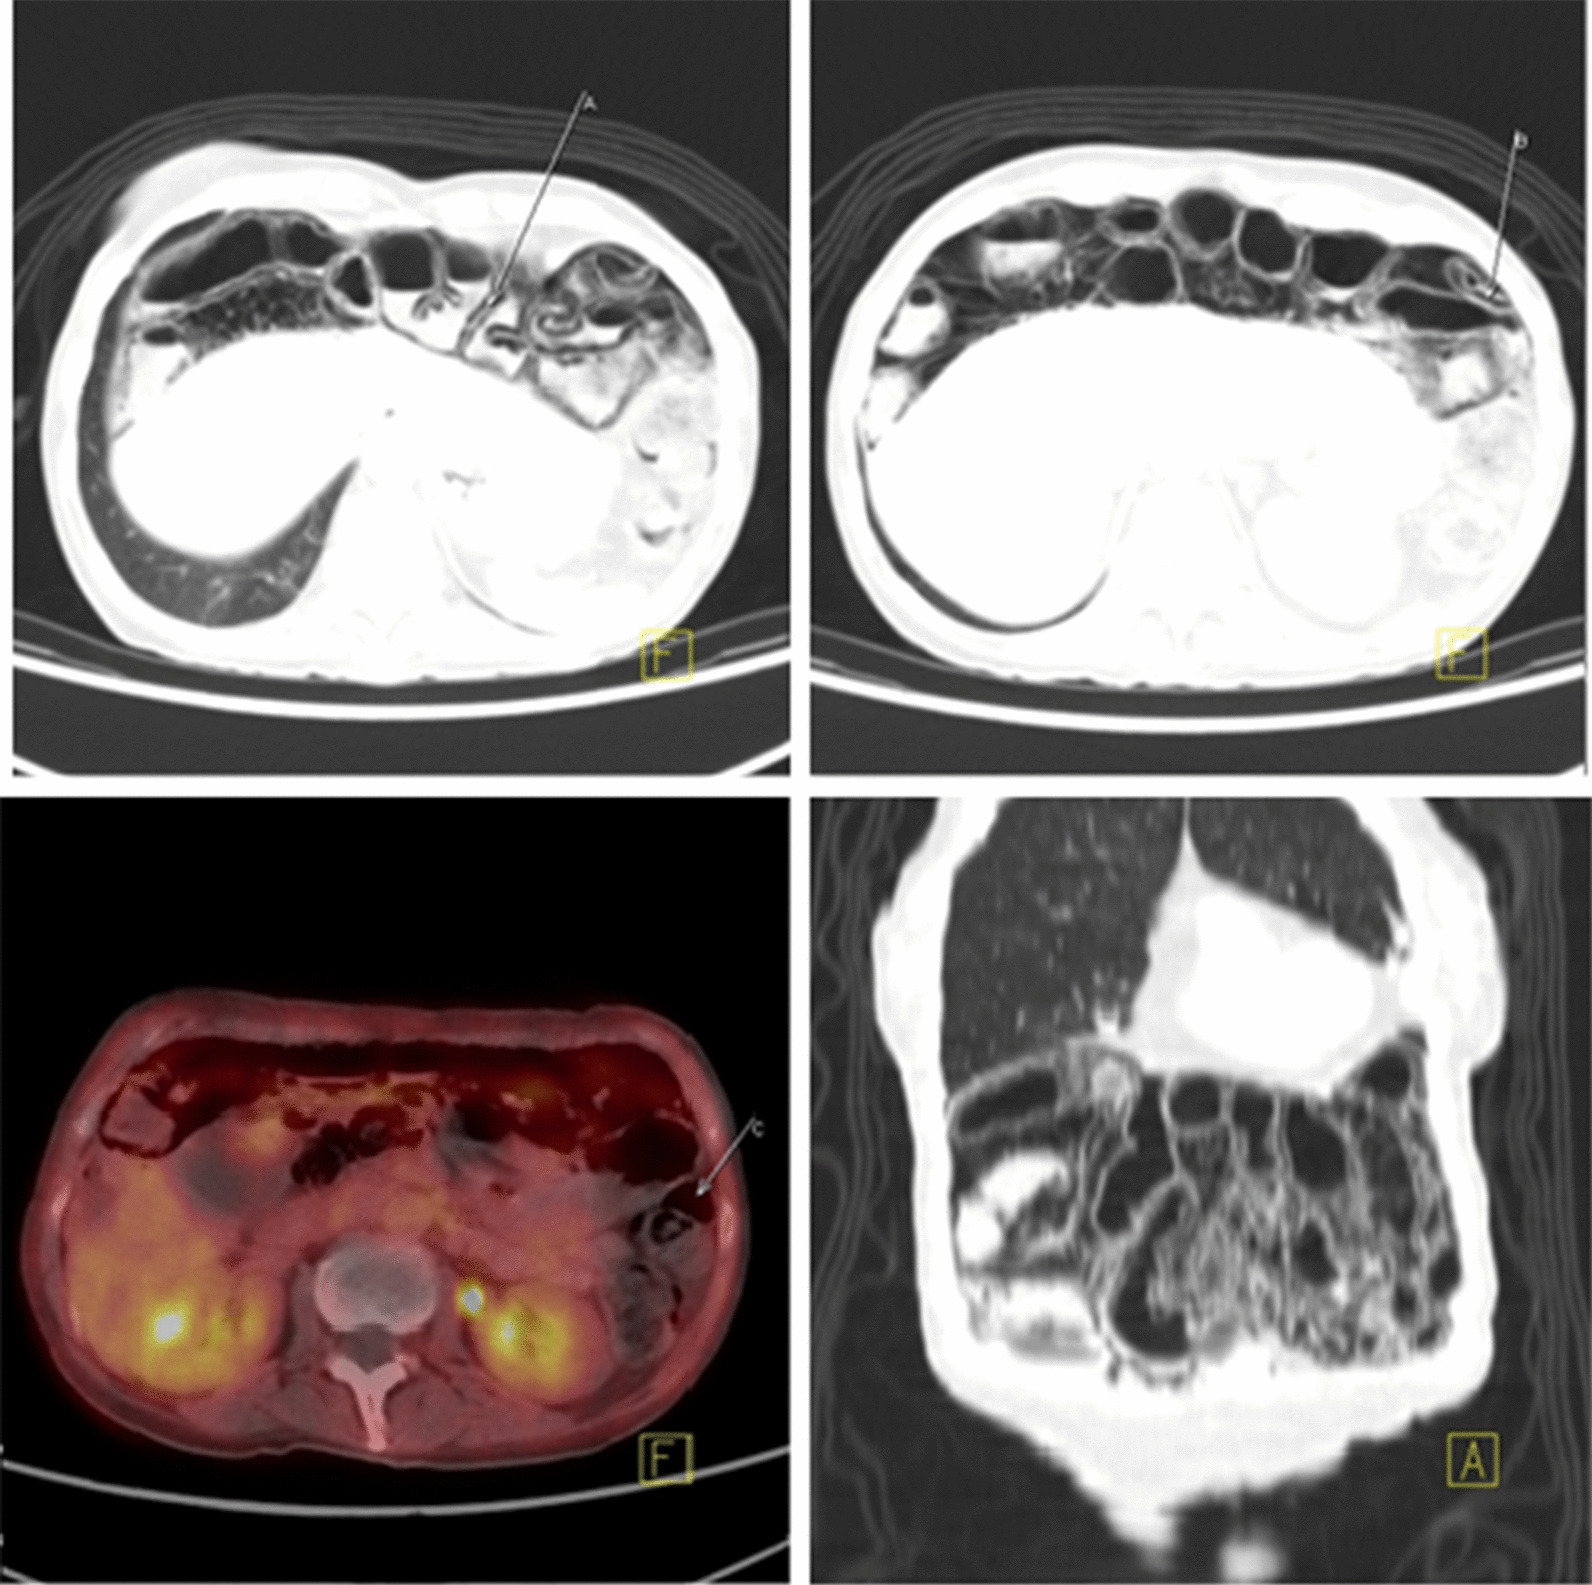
Positron emission tomography–computed tomography January 2019. (Images a and b show radiological signs of PI with mild sign of pneumoperitoneum showed in image c.)
